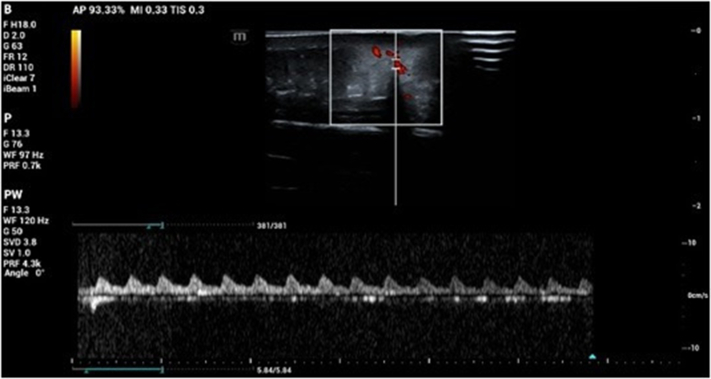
High-frequency ultrasound: Doppler spectral showing low-velocity arterial flow.
